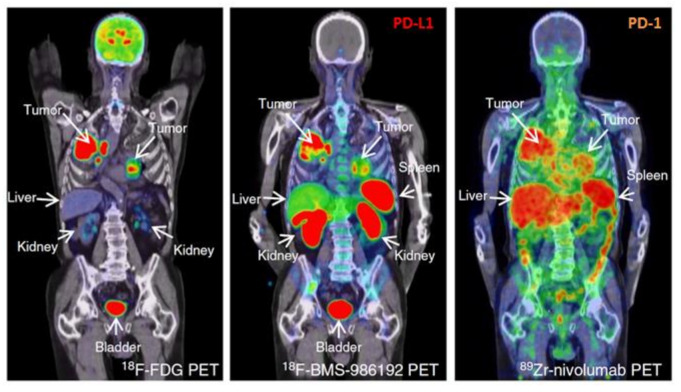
Comparison of [^18^F]FDG PET/CT with anti-PD-L1 (18F-BMS-986192) and anti-PD-1 (89Zr-labeled Nivolumab) immuno-PET images in the same patient with NSCLC. Along the high glucose metabolism of the tumor in both lungs and mediastinal lymph nodes, a heterogeneous tracer uptake for 18F-BMS-986192 PET/CT and 89Zr-labeled Nivolumab PET/CT within and between tumors is demonstrated. Modified from Niemeijer AN et al. Whole body PD-1 and PD-L1 positron emission tomography in patients with non-small-cell lung cancer. Nat Commun 2018;9:4664. [106]; Licensed under a Creative Commons license: http://creativecommons.org/licenses/by/4.0/) Last access date: 2 November 2021.
